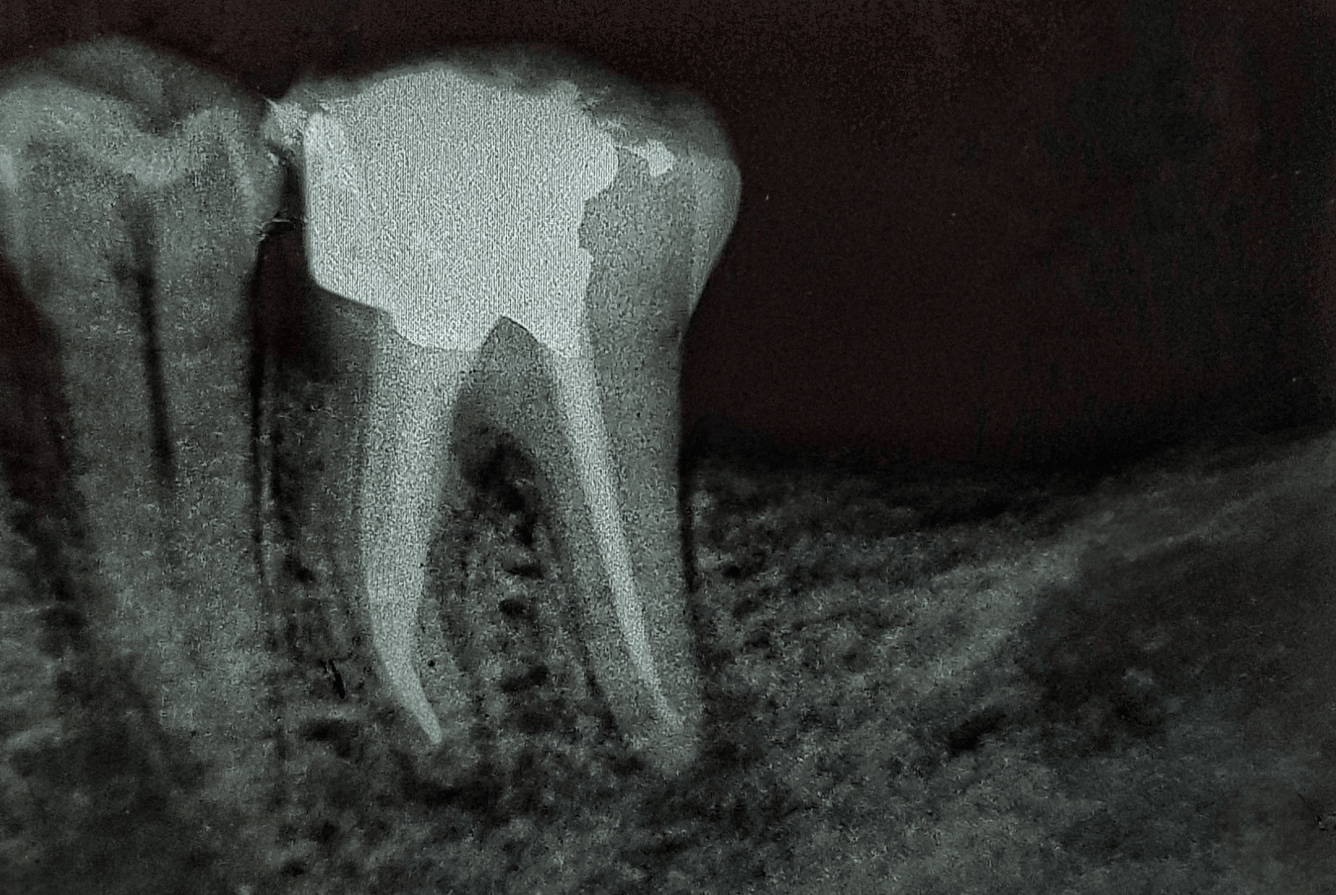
Post-obturation radiograph Postoperative radiograph after obturation and post-endodontic restoration of the treated tooth.
